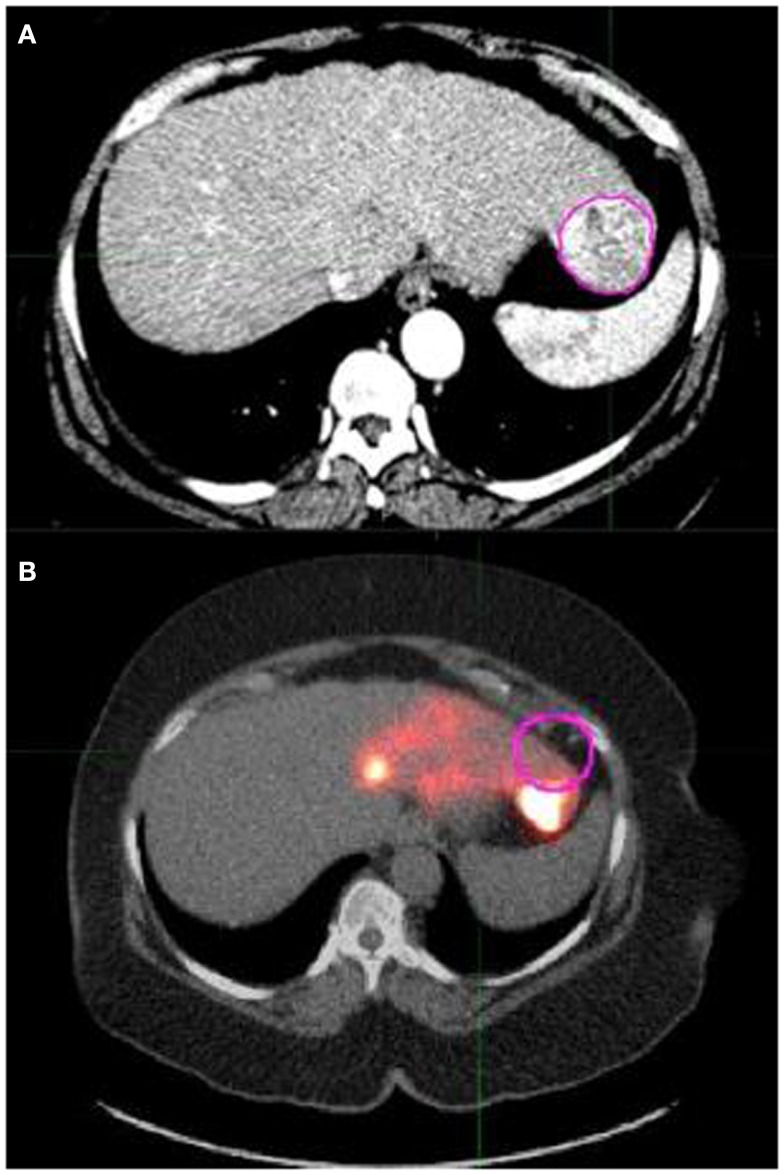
(A) shows a pink contour drawn around a tumor in an axial slice of the arterial phase abdominal CT scan. (B) shows the same tumor contour overlaid on the AC CT/PET fusion image that was acquired following left lobe ^90^Y microsphere therapy. (B) exhibits that contours drawn on the arterial CT show poor overlap with the corresponding region in the AC CT/PET fusion image. This can lead to inaccurate activity concentration determinations and necessitated the need to deform the arterial CT scan for more accurate contour statistic calculations.
